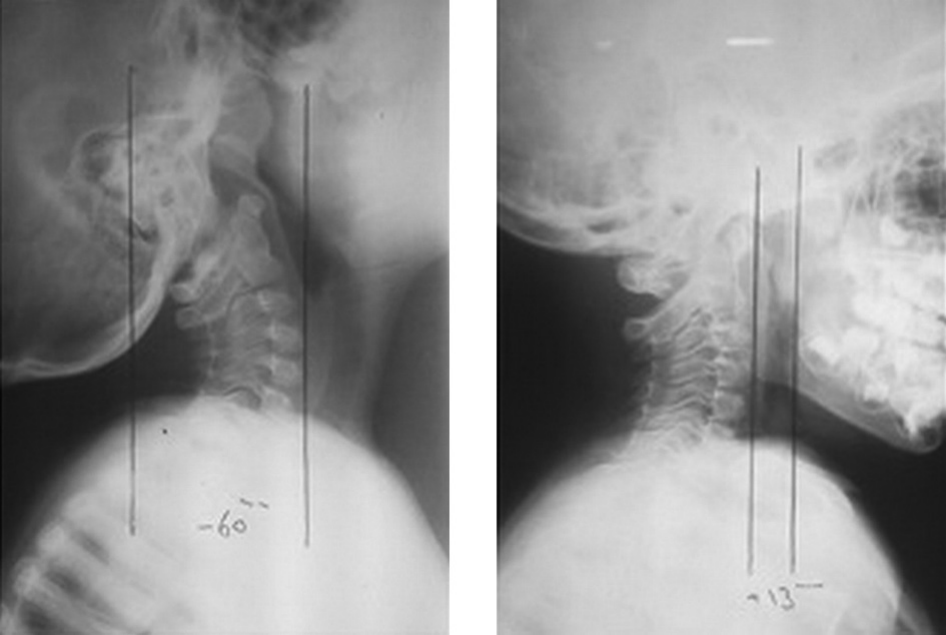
Forward Head Posture, When Measured From the Sella Turcica Down to the Anterior Portion of the C4 Disc. Preoperative and postoperative X-ray.
